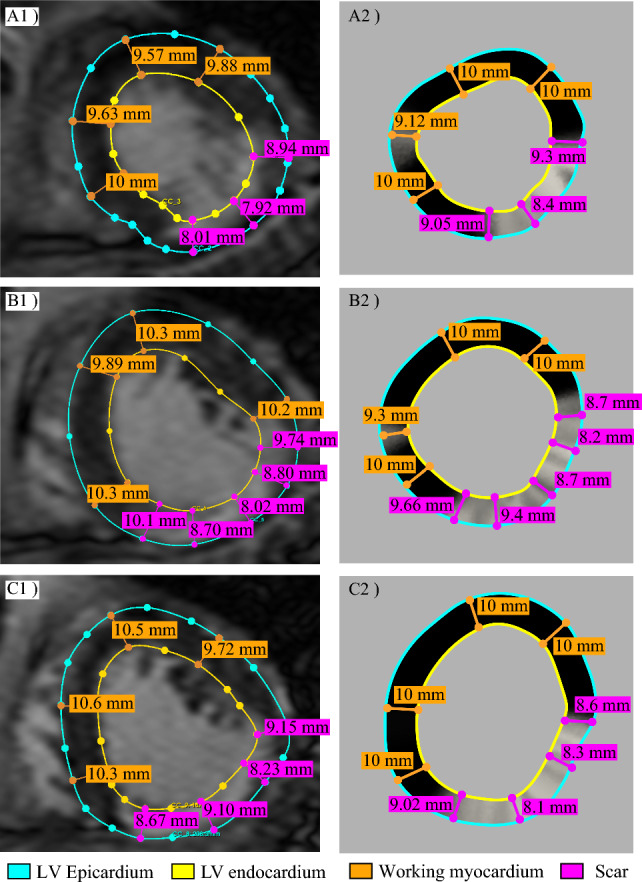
Comparision between MRI slices acquired in vivo vs geometry slices obtained in silico for (A1, A2) distal, (B1, B2) medial and (C1, C2) proximal portion of the ventricle in the long-axis direction. Measuring lines are reported in magenta for the scar region and in orange for the working myocardium, respectively
